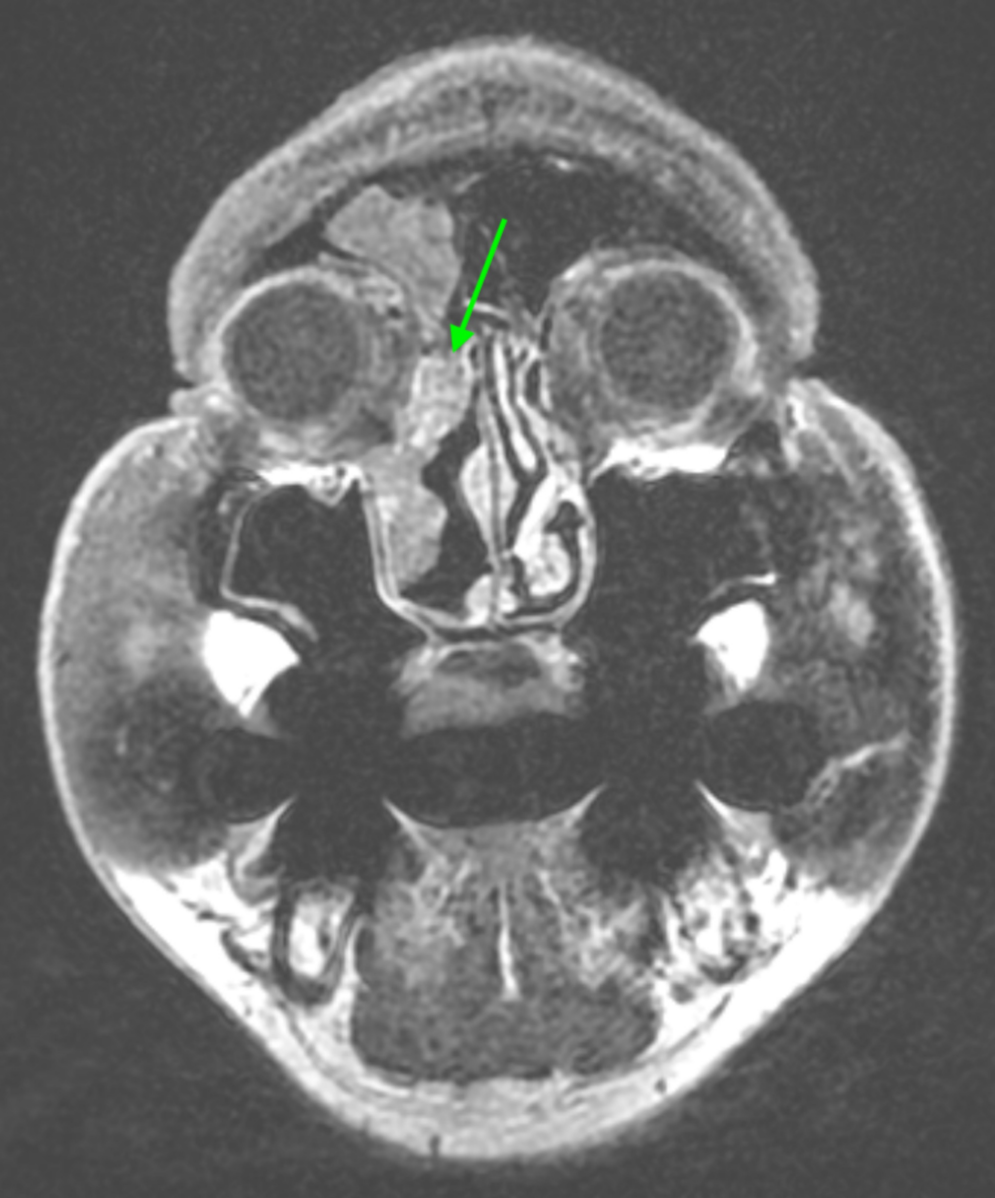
MRI showing recurrent tumor extending from the frontoethmoidal recess, along the lateral nasal wall.
